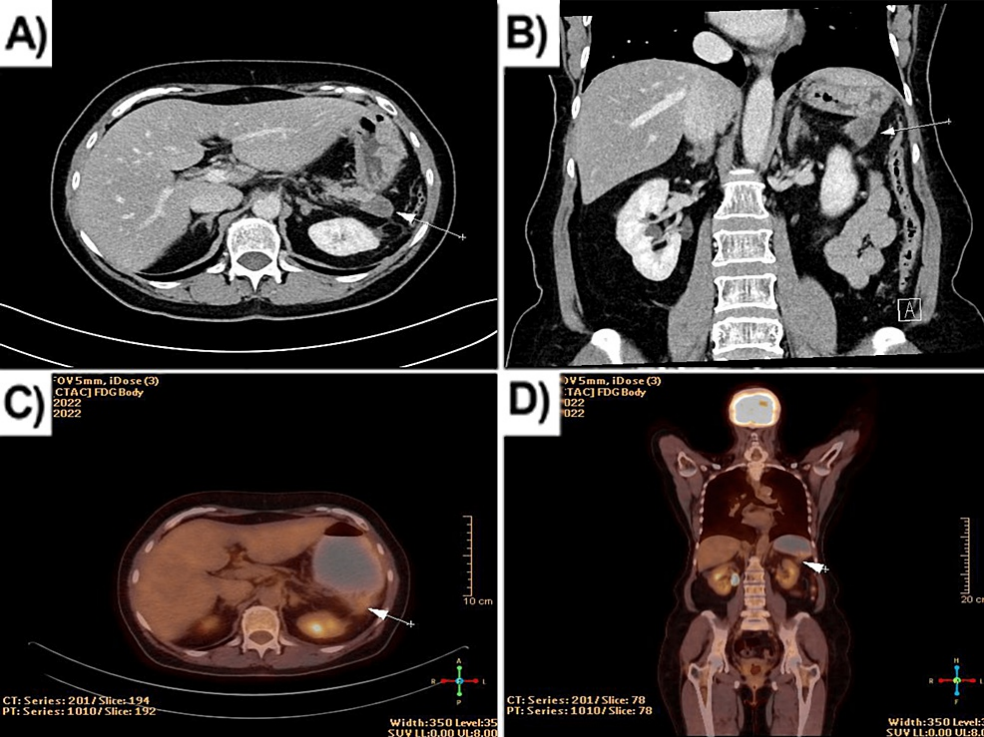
Image tests (five months after the operation). (A) Abdominal computed tomography (axial plane) showing a mass suspicious for recurrence; (B) Abdominal computed tomography (coronal plane); (C) PET/CT (axial plane); (D) PET/CT (coronal plane). PET/CT: positron emission tomography/computed tomography
